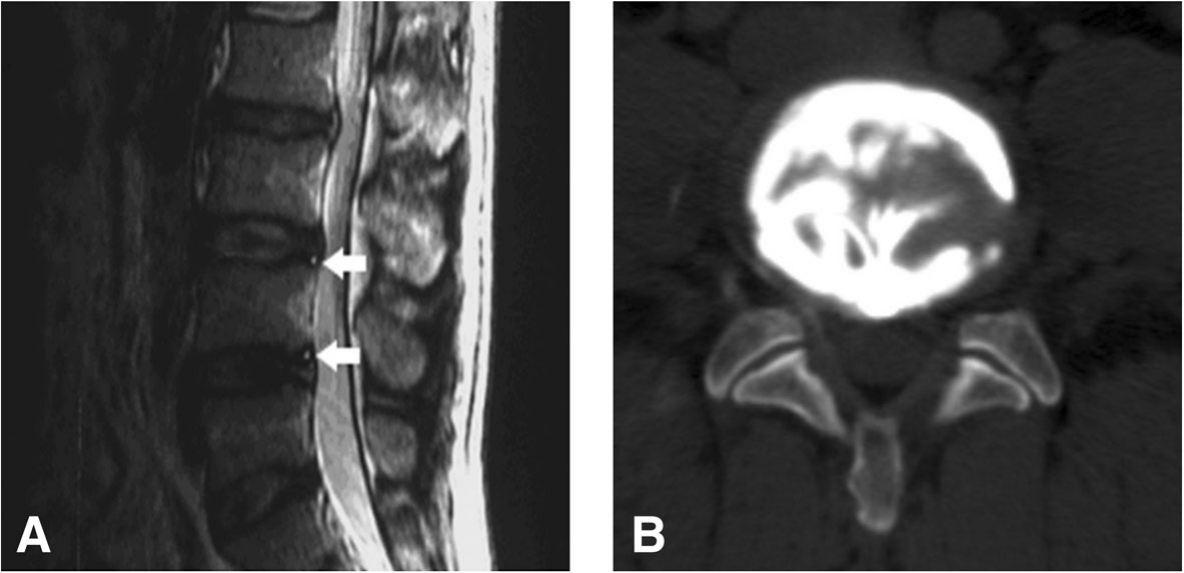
A 46-year-old man with low back pain for 9 months. a Sagittal T2-weighted MR image of the lumbar spine shows two HIZs at L3/4 and L4/5 (white arrow). b Axial post-discography CT image shows a grade 4 annular disruption at L3/4. Discography produced concordant pain
